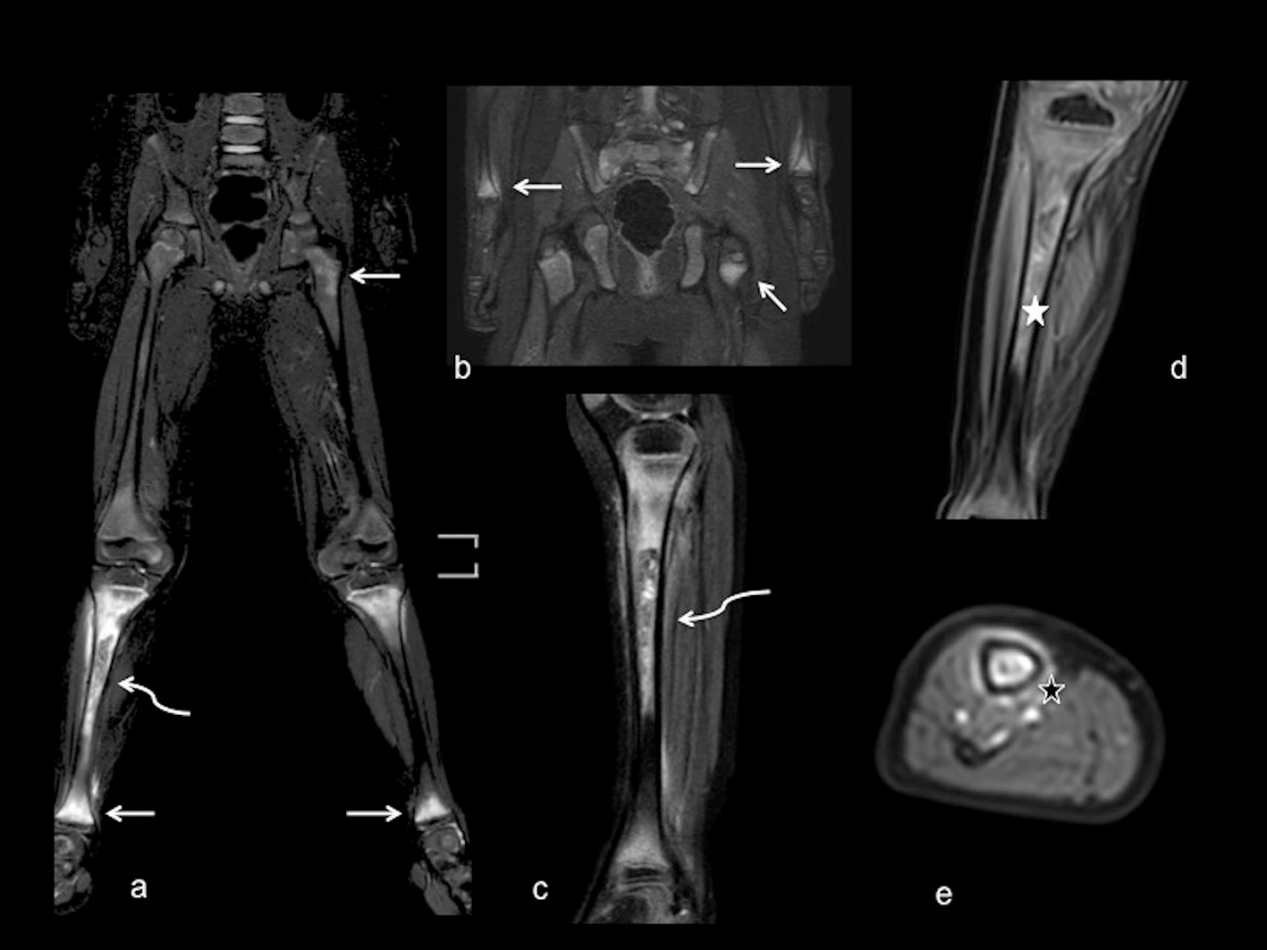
MRI with large field of view. Coronal (a,b) and sagittal (c) T2-weighted fat saturated views demonstrate abnormal marrow signal of femoral, tibia and wrist metaphyses (white arrows) bilaterally, with more evident involvement of right tibia diaphysis, showing abnormal inhomogeneous signal intensity (curved arrows). Coronal and axial views (d,e) after contrast administration show inhomogeneous enhancement of bone marrow (white star) and periosteal tissue (black star), without periosteal collections.
